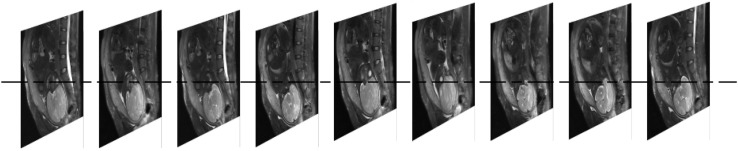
Each patient contains 9 layers of MRI sequence images.
